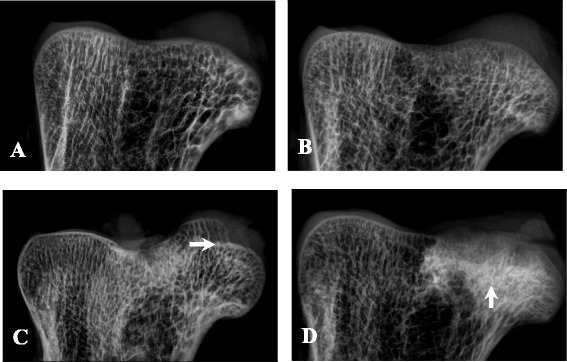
The radiographic imaging findings. a The X-ray imaging of normal ostrich femur head. b The X-ray imaging of ostrich femur head at postoperative week 6: no significant changes were observed in the femoral head contour or bone density. c The X-ray imaging of ostrich femur head at postoperative week 12: alterations in the femoral head contour (*arrow*) and decrease in the height of the femoral head. d The X-ray imaging of the collapsed ostrich femur head: the collapsed femoral head and high-density shadows at the center of the femoral head (*arrow*)
